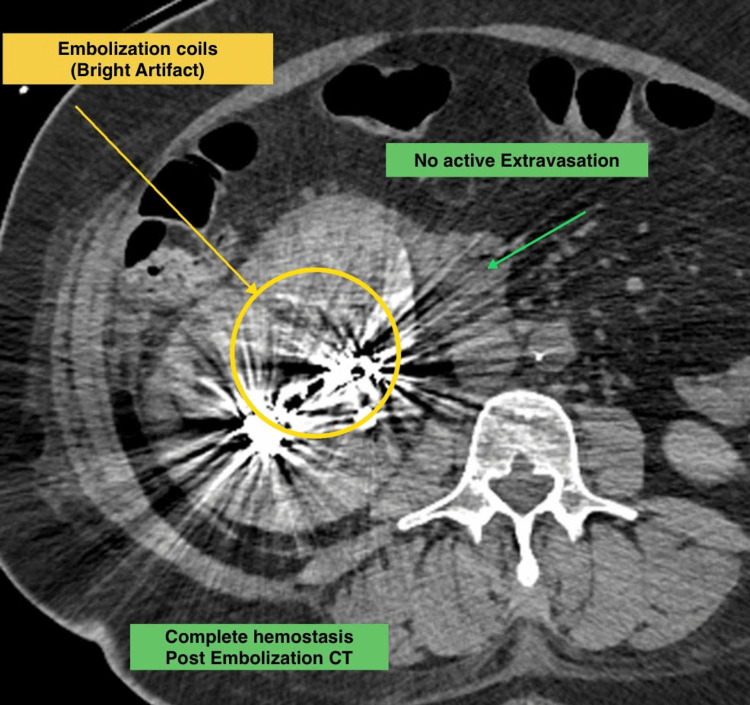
Axial post-embolization contrast-enhanced CT image showing multiple embolization coils (bright artifacts) within the right kidney at the site of the treated angiomyolipoma No active contrast extravasation is identified, indicating complete hemostasis.
